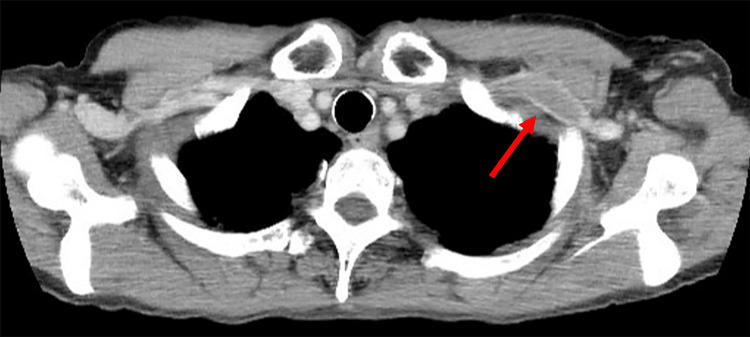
Contrast-enhanced CT. Contrast-enhanced axial CT of the upper chest showing a filling defect in the left subclavian vein (red arrow), consistent with thrombosis.
